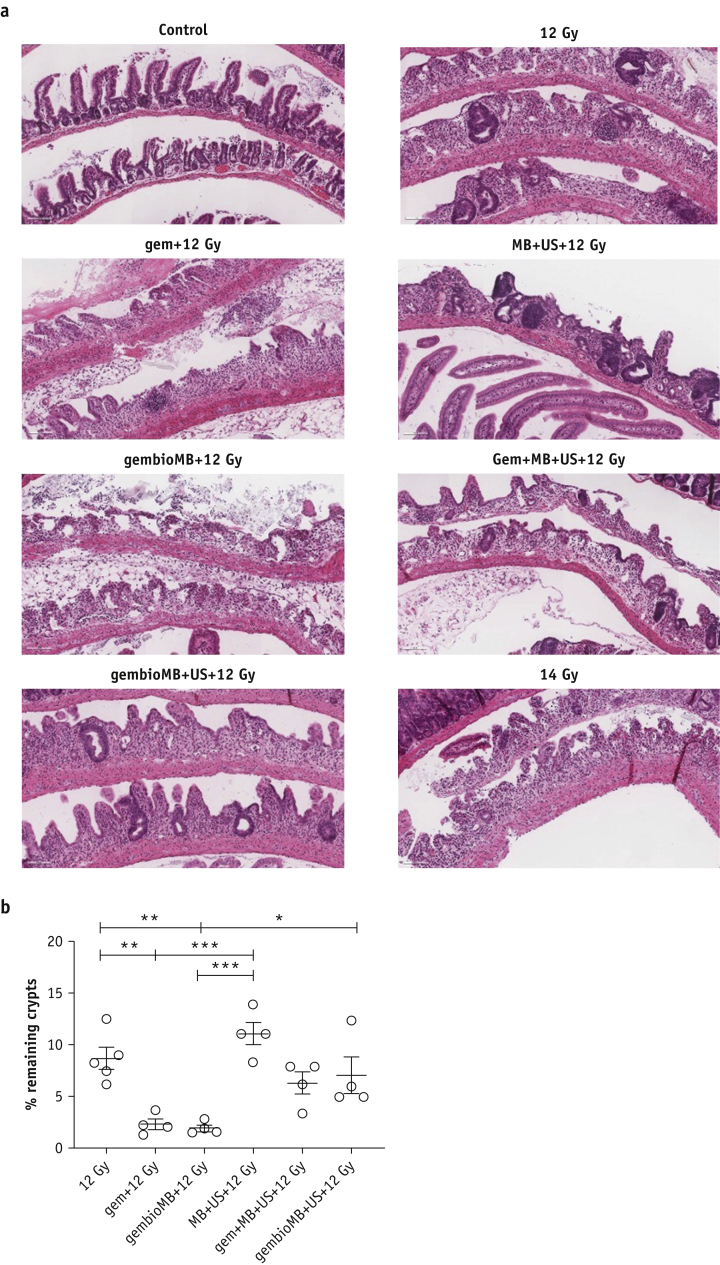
Acute normal tissue toxicity. (a) Representative images of intestinal crypt from each treatment group. Scale bar represents 100 μm. (b) Assessment of acute normal tissue toxicity using crypt survival. Conventional chemoradiation therapy (gem + 12 Gy) or gembioMB + 12 Gy resulted in significant reduction in crypt survival compared with IR at 12 Gy only. There was no difference between 12 Gy and the 3 ultrasound-mediated drug delivery methods (MB + US + 12 Gy, gem + MB + US + 12 Gy, and gembioMB + US + 12 Gy), indicating that exacerbation of normal-tissue toxicity by addition of chemotherapy could be avoided; ∗*P* < .05, ∗∗*P* < .01, ∗∗∗*P* < .001. n = 5 for 12 Gy, and n = 4 for all of the other groups. *Abbreviations:* gem = gemcitabine; gembioMB = gemcitabine-conjugated microbubbles; IR = radiation; MB = microbubbles; US = ultrasound.
